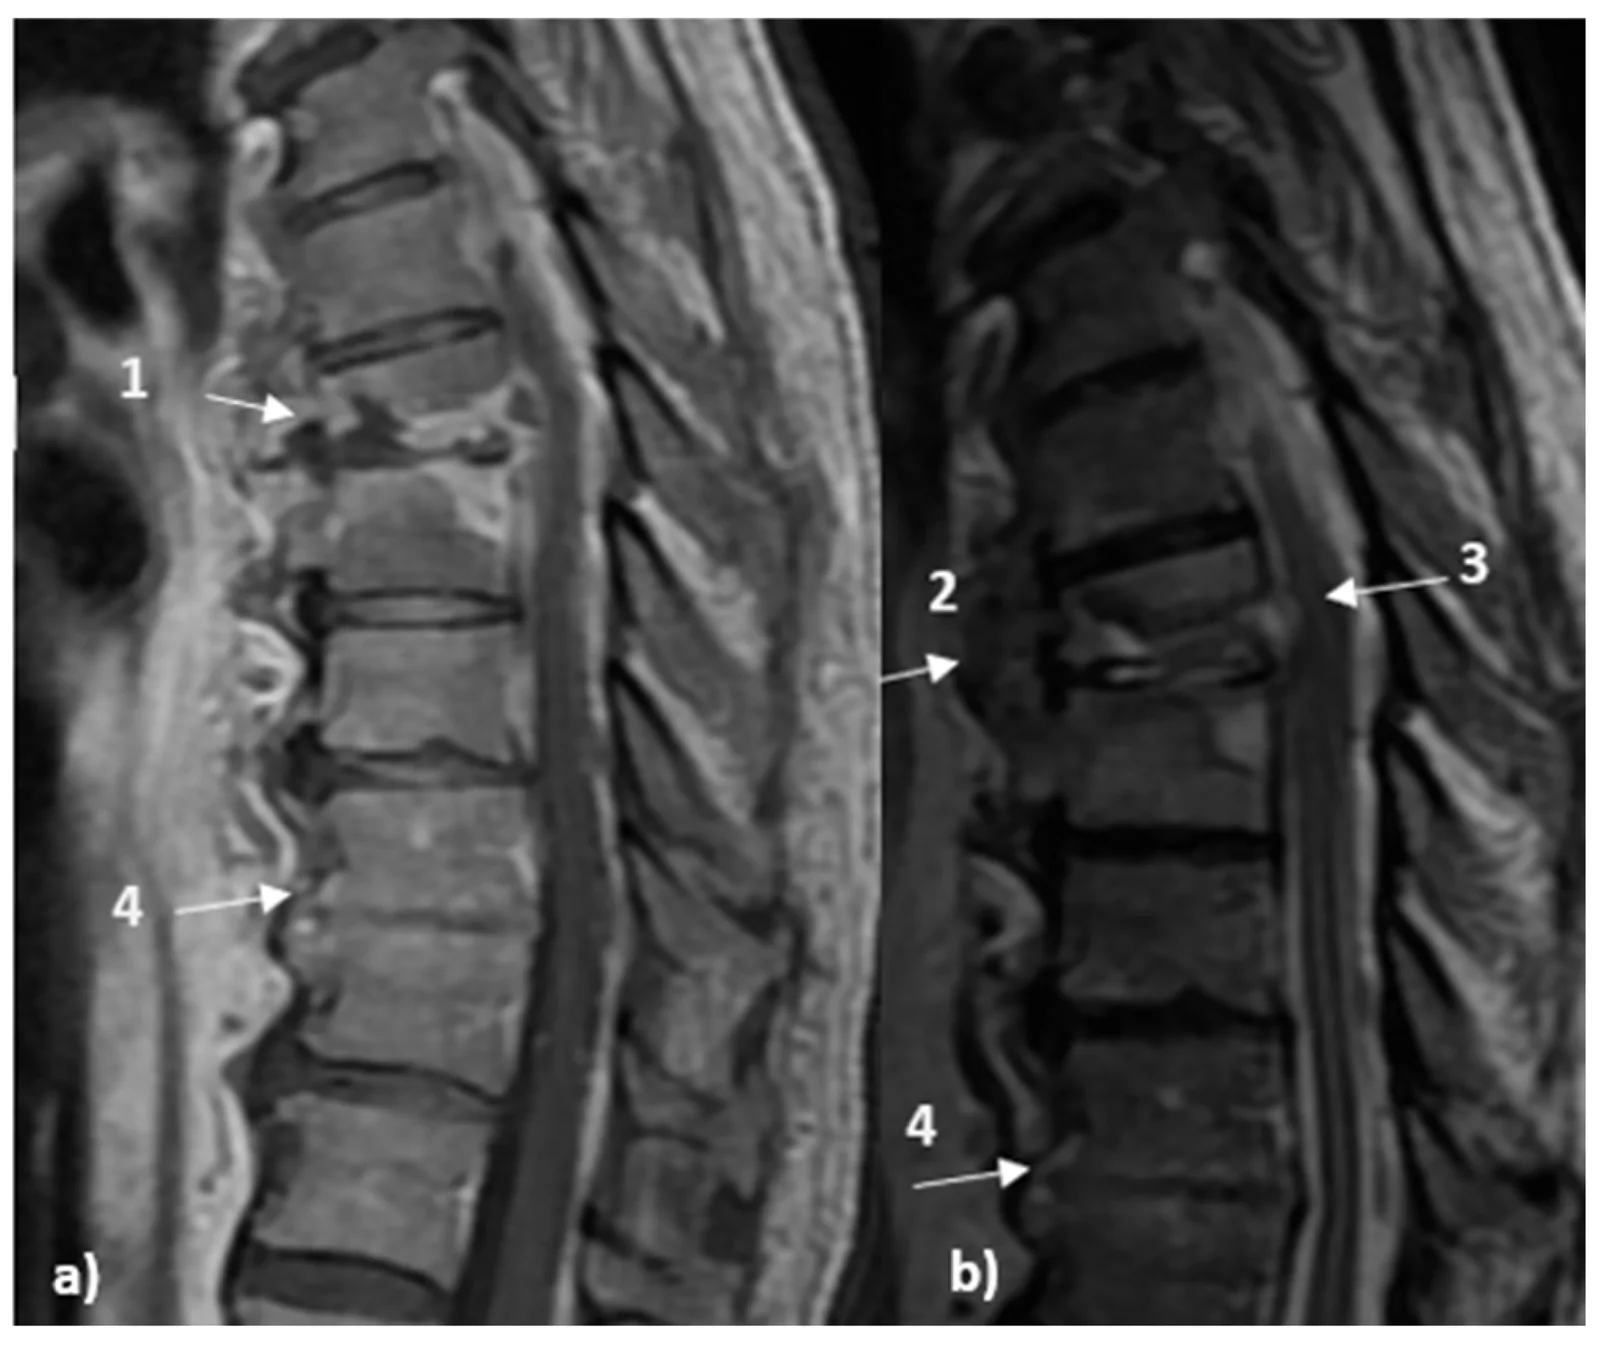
Tuberculous pattern with non-contiguous disease: skip lesions and multilevel extension. Sagittal post-contrast T1-weighted (a) and T2-weighted (b) images show T7–T8 destruction with anterior collapse (1), heterogeneous enhancement, paravertebral (2) and epidural abscesses (3), and a second non-contiguous level at T10–T11 (4), a distribution that favors TS.
